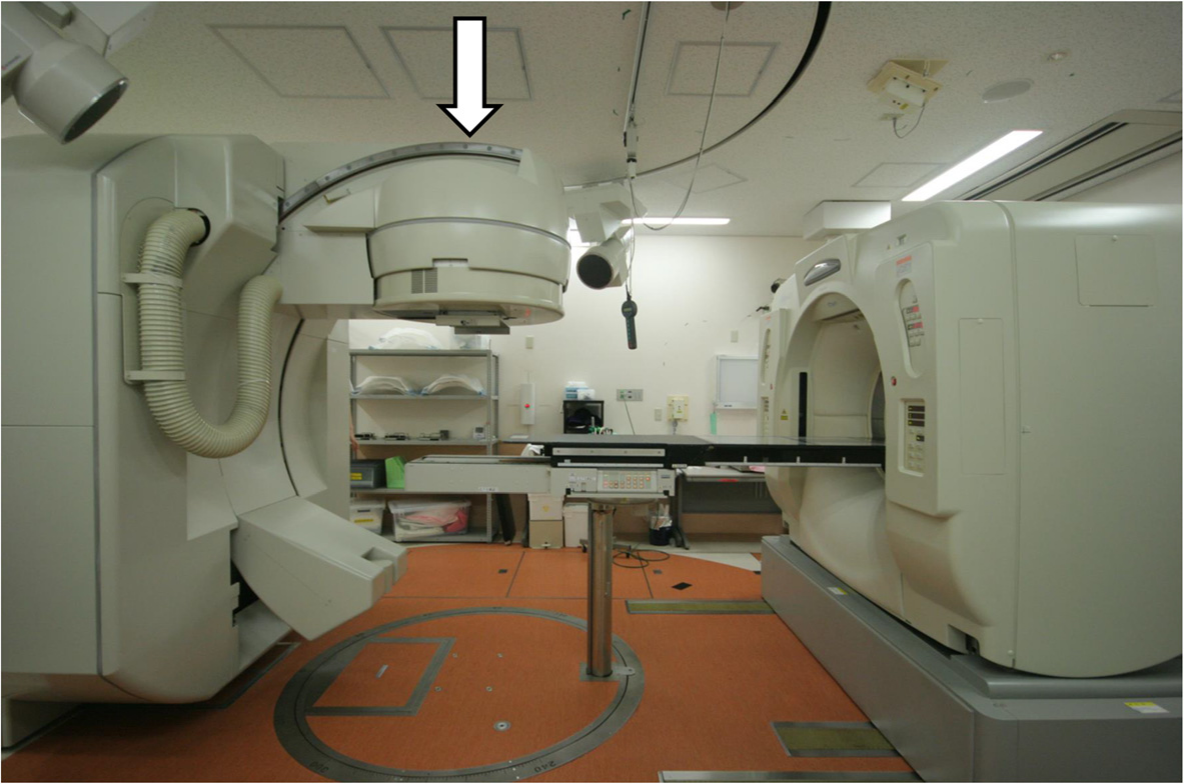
The CT-linac integrated radiation system at Sagamihara Kyodo Hospital. The C-arm mounted linac is indicated by an arrow
